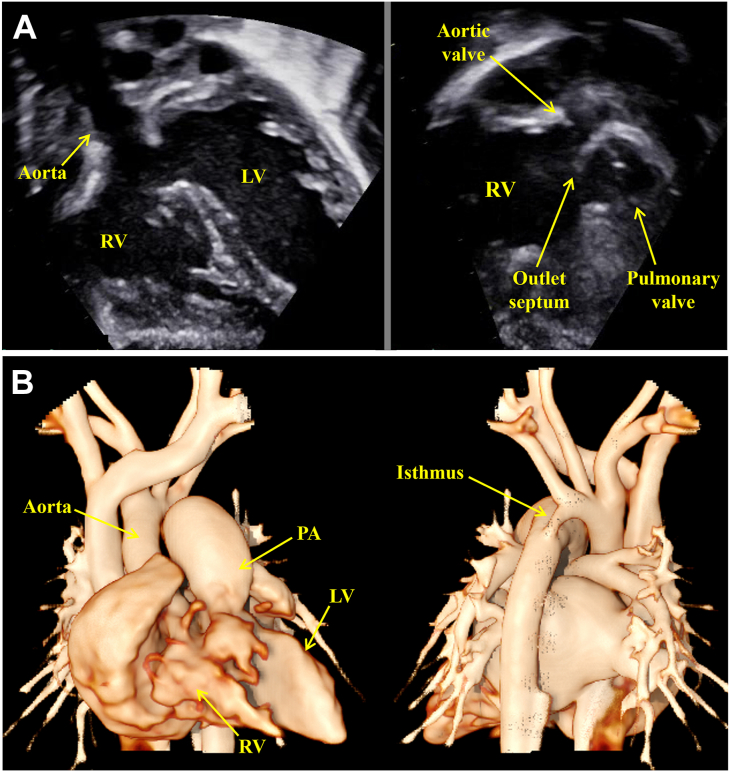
(A) Preoperative transthoracic echocardiography. (B) Preoperative 3-dimensional computed tomographic images. (LV, left ventricle; PA, pulmonary artery; RV, right ventricle.)
